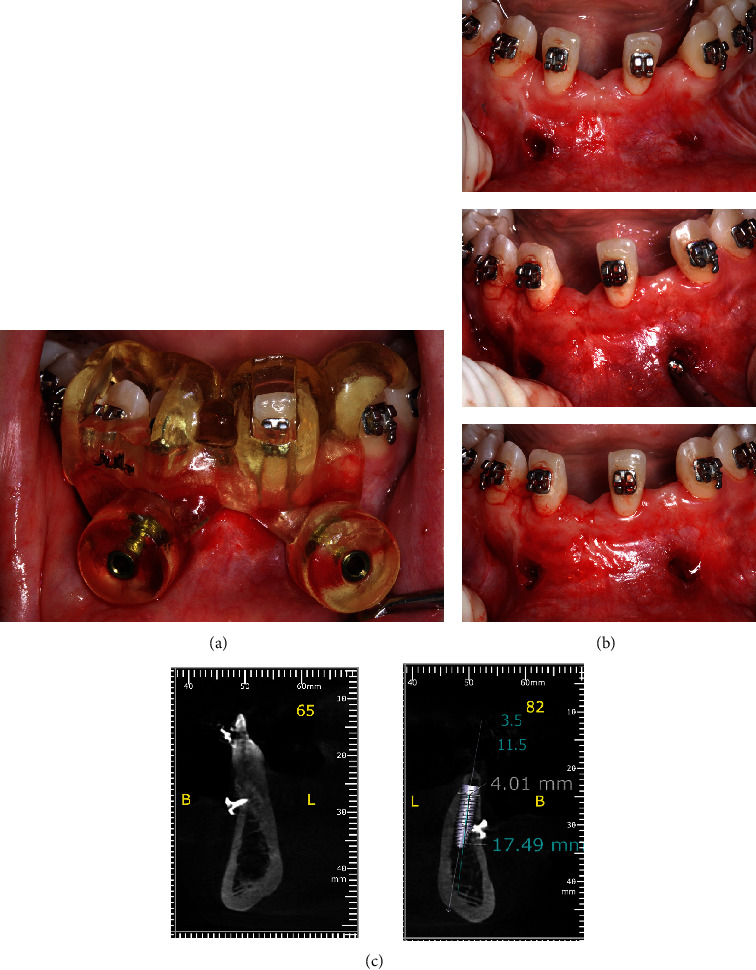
(a) Surgical guide positioned for implant placement; (b) removal of a miniscrew prior to implant placement for subsequent imaging examination; and (c) CBCT imaging revealing the width of the alveolar crest allowing the placement of a proper diameter implant.
